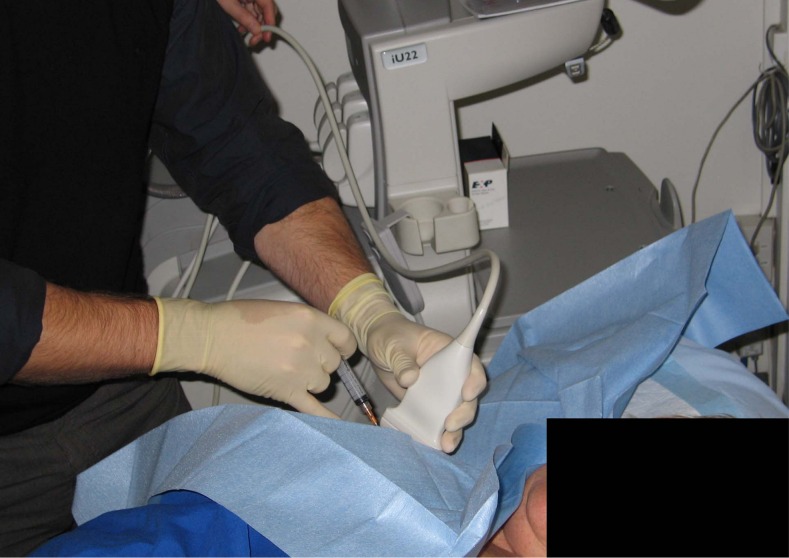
Ultrasound guided diagnostic injection into the subacromial bursa
